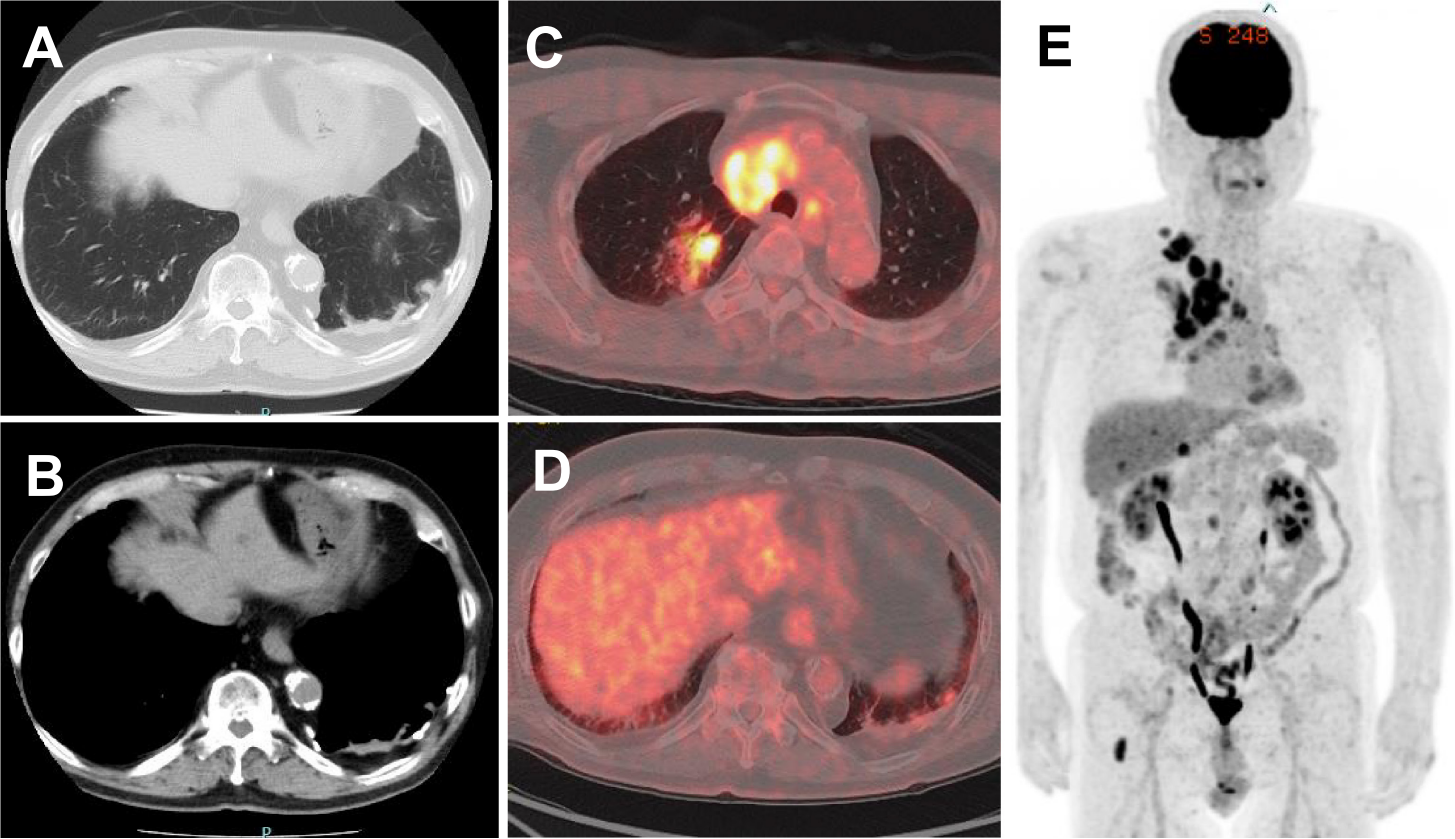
Computed tomography shows a partially calcified pleural plaque and left lower lobe subpleural rounded atelectasis (A and B). Positron emission tomography revealed that ^18^F‐fluorodeoxyglucose uptake was avid only in the right upper lobe nodule and not in the rounded atelectasis (C–E).
